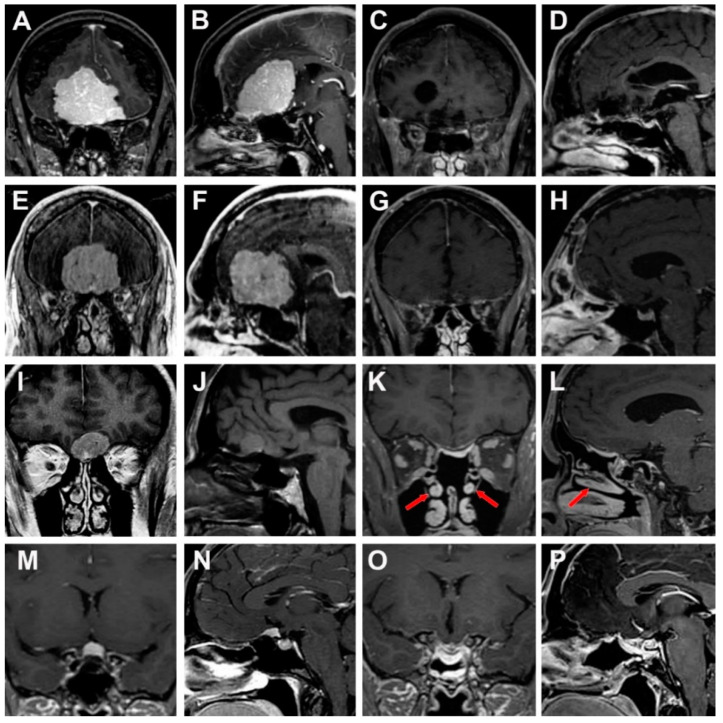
Preoperative and postoperative magnetic resonance imagines (MRI) of the clinical cases. (A–D) Case 1, preoperative coronal and sagittal view showing an anterior skull base meningioma centered over the planum sphenoidale (A,B). Postoperative coronal and sagittal view confirming gross total resection (C,D). (E–H) Case 2, preoperative coronal and sagittal section of olfactory groove meningioma (E,F) and postoperative coronal and sagittal view documented the gross total resection of the lesion (G,H) (I–L) Case 3, preoperative coronal and sagittal view of olfactory groove meningioma (I,J). Postoperative MRI after endoscopic transnasal superior ethmoidal approach with preservation of the middle turbinate (red arrow) (K,L). (M–P) Case 4, preoperative coronal and sagittal section of tuberculum sellae meningioma (M,N) and postoperative MRI after endoscopic endonasal transplanum approach (O,P).
